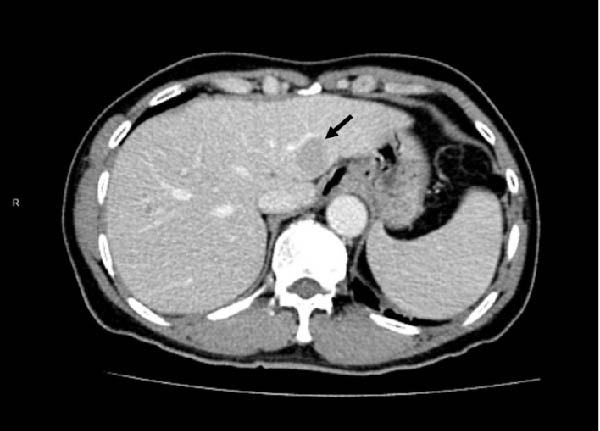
CT showing a segment 2/3 tumor (arrow).
